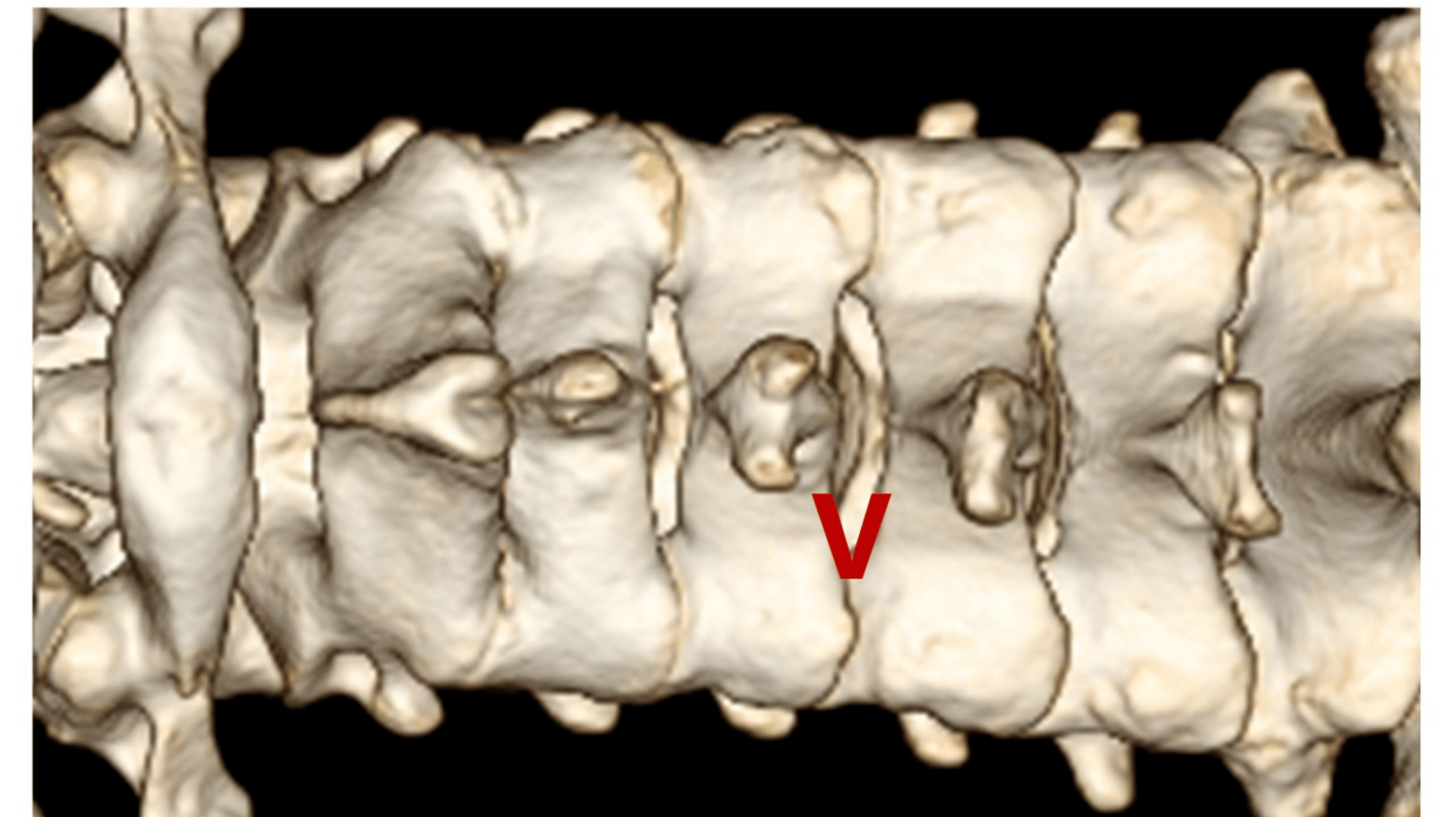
Preoperative 3D-CT image The overlap of the superior and inferior articular processes enabled the visualization of the so-called Interlaminar V CT: computed tomography
